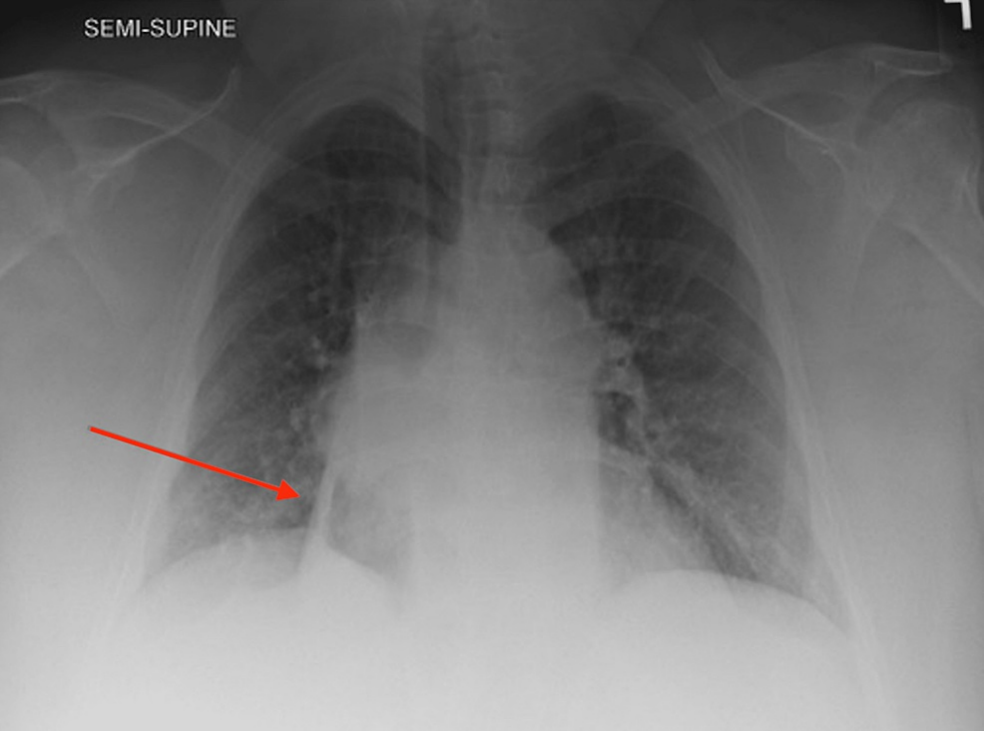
Chest X-ray revealing right lower lobe opacity concerning for lung mass (red arrow)
